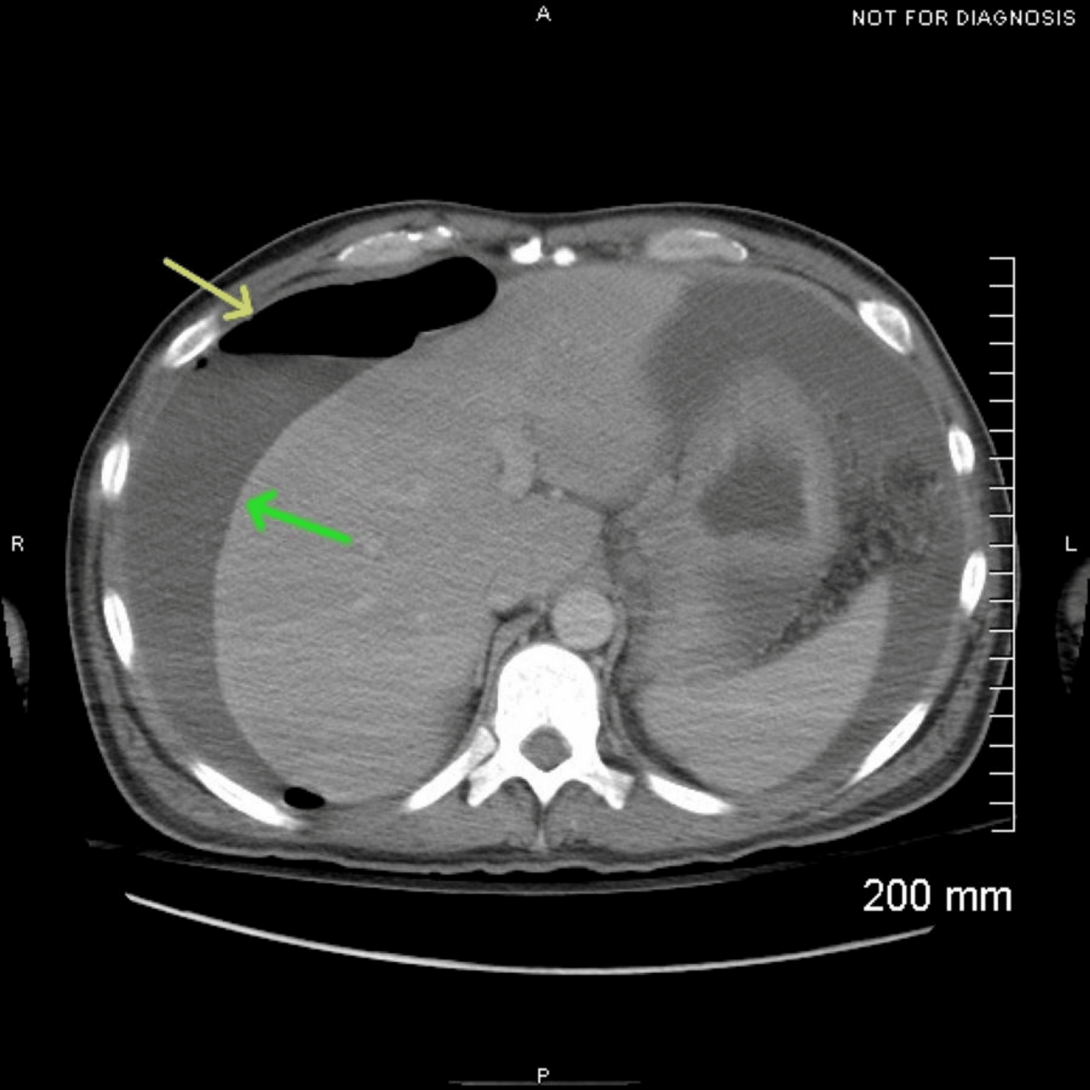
Perihepatic pneumoperitoneum with ascites on CT Axial contrast-enhanced CT demonstrating pneumoperitoneum (yellow arrow) with adjacent perihepatic ascites (green arrow). CT: computed tomography
